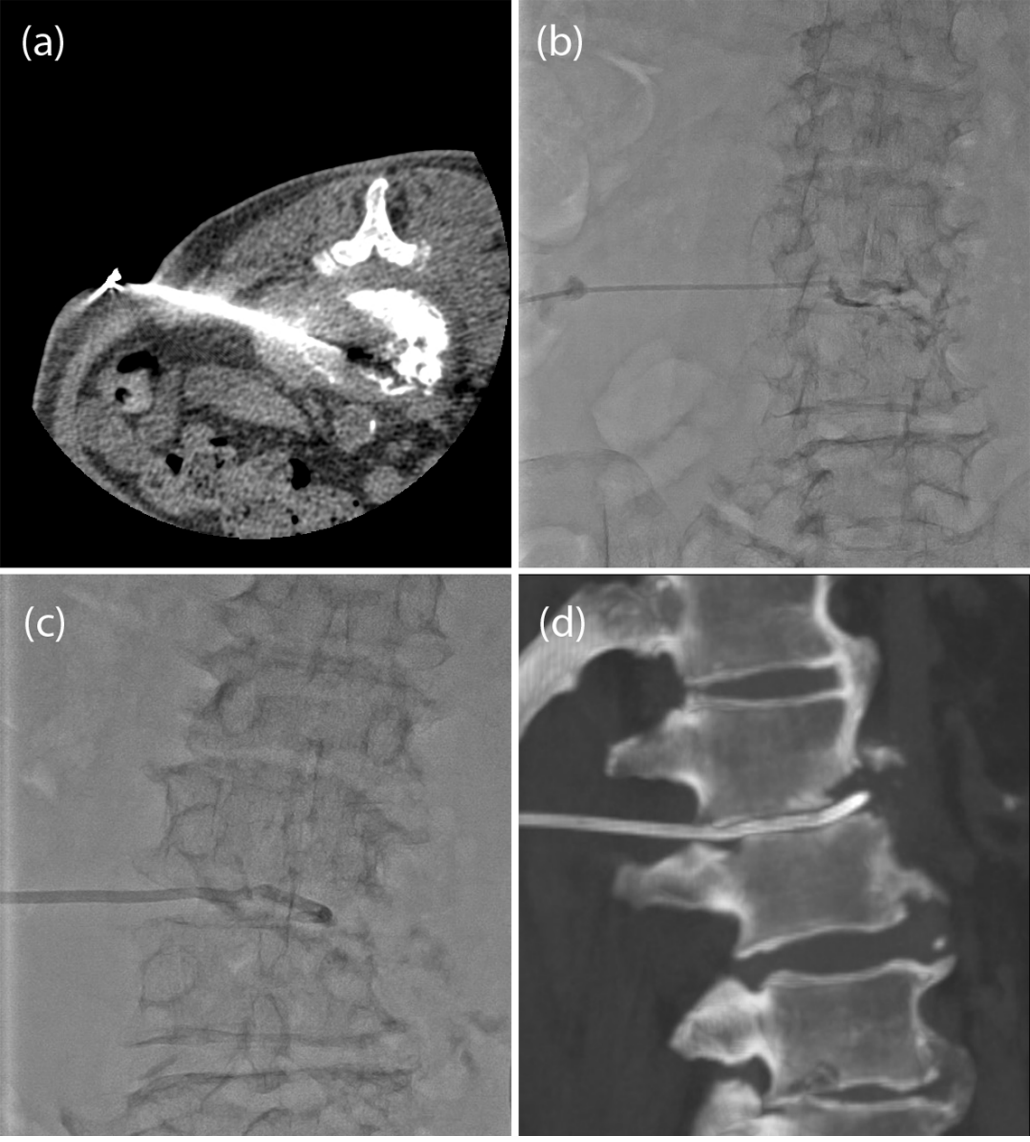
Patients who underwent intradiscal and intrapsoas muscle drainage tube placement via CT-guided percutaneous insertion. (a) CT image showing the procedure for creating puncture to the disc space using a 18G Surflo needle guided by CT in the prone position under local anesthesia with procaine. (b) Fluoroscopy image demonstrating the injection of contrast-enhanced material through the outer sheath of the Surflo needle and then pulling it out. (c) Fluoroscopy image demonstrating a drainage tube being inserted into the discal space. A stiff-type guidewire (Amplatz ultrastiff guidewire, COOK medical Japan, Tokyo, Japan) was used. (d) A reconstructed CT image after the procedure showing that the drainage tube was located in the discal space.
